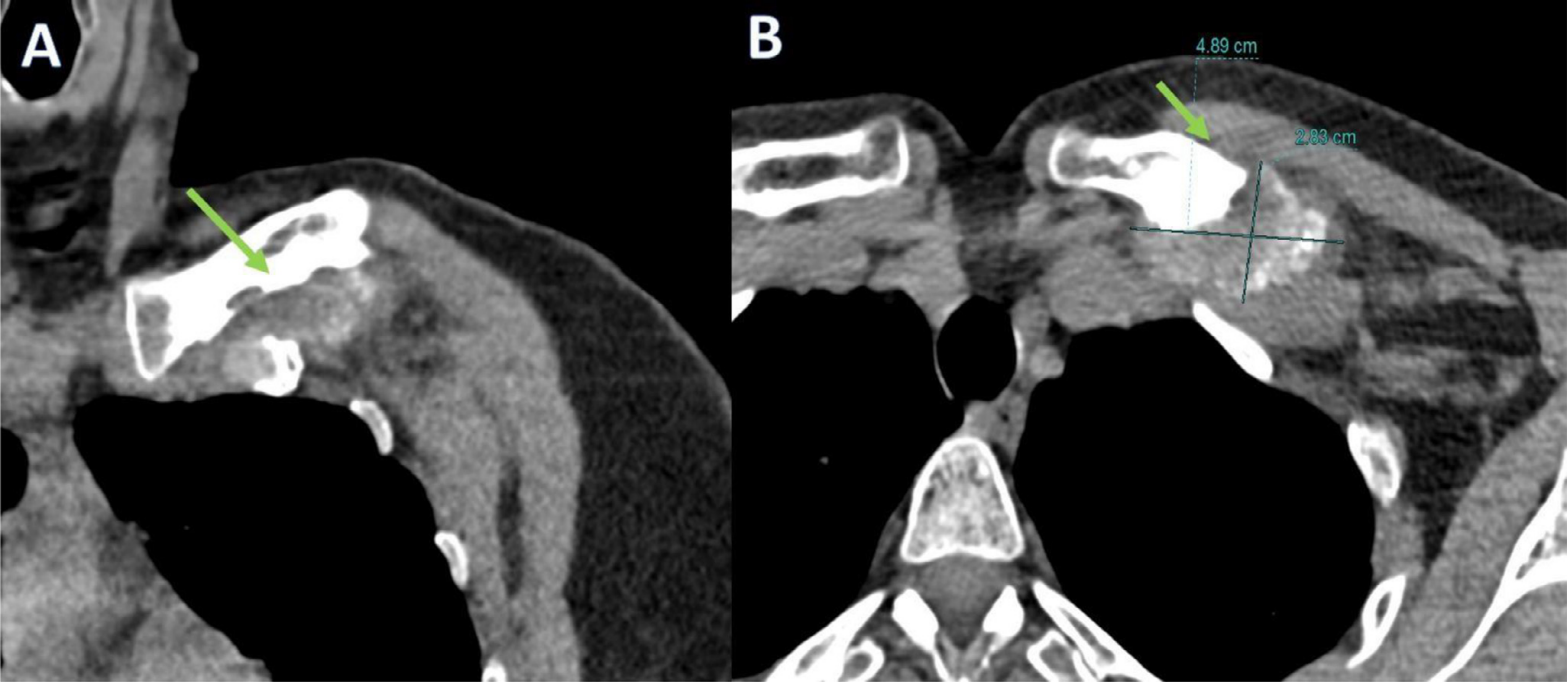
Volumetric computed tomography of the left upper thoracic area in the coronal (A) and axial (B) planes detecting soft tissue lesion with chondroid calcifications, between the 1st rib, its chondrocostal joint, and the clavicle associated with new bone formation and erosions of the inferior clavicular margin (green arrows).
